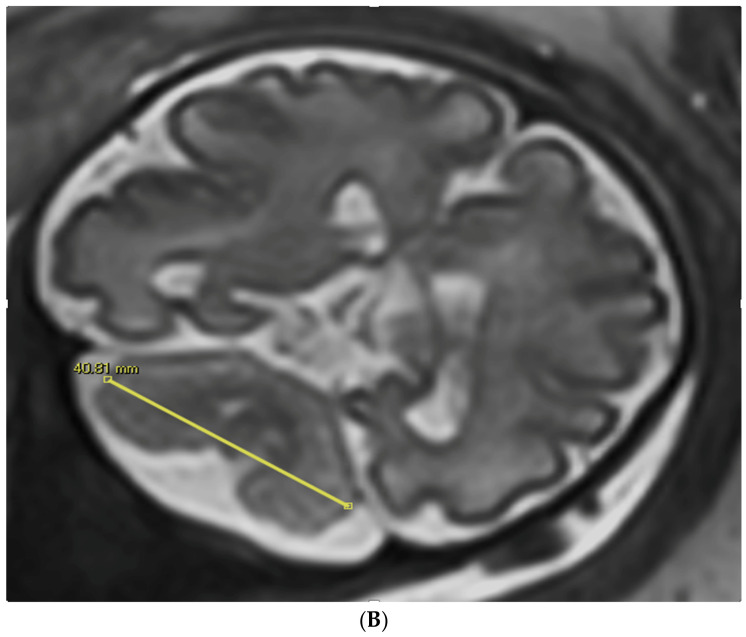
TCD measured in an image by trans-abdominal axial US scan at 31 weeks of gestation (A) and by coronal MRI scan at 32 weeks of gestation (B).
